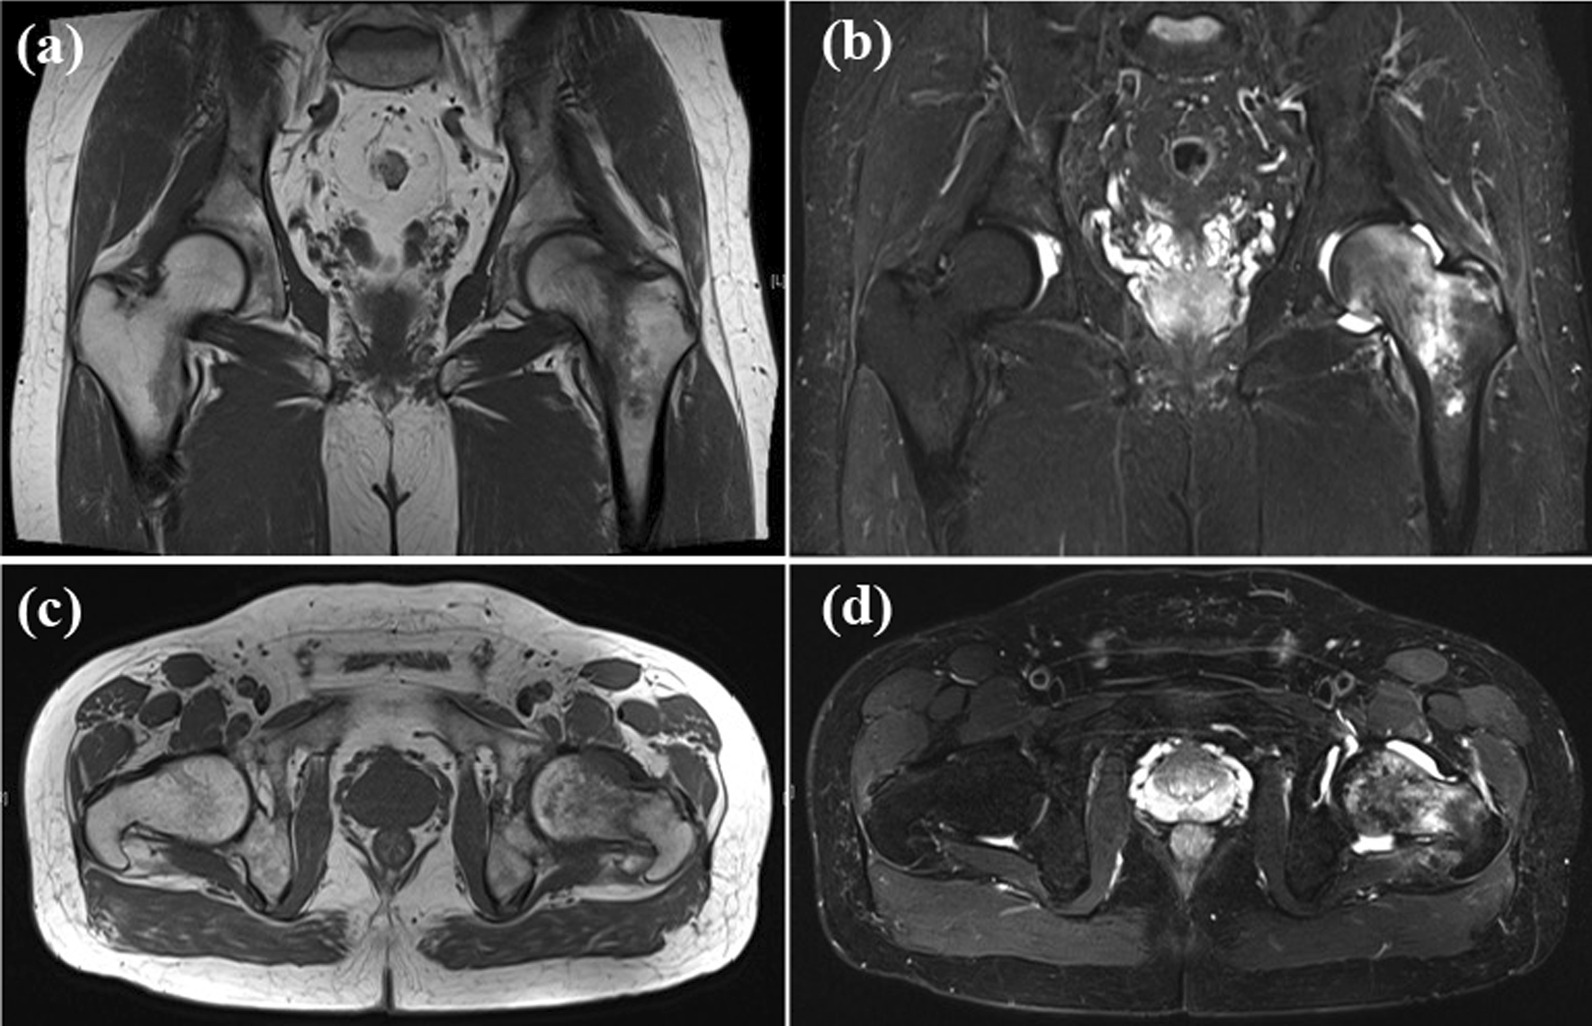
Images taken 3 months after the injury. Coronal view (a) and axial view (c) of hip joint T1-weighted MRI, and coronal view (b) and axial view (d) of hip joint short tau inversion recovery MRI. A fracture line with bone marrow edema was found in the left proximal femur
